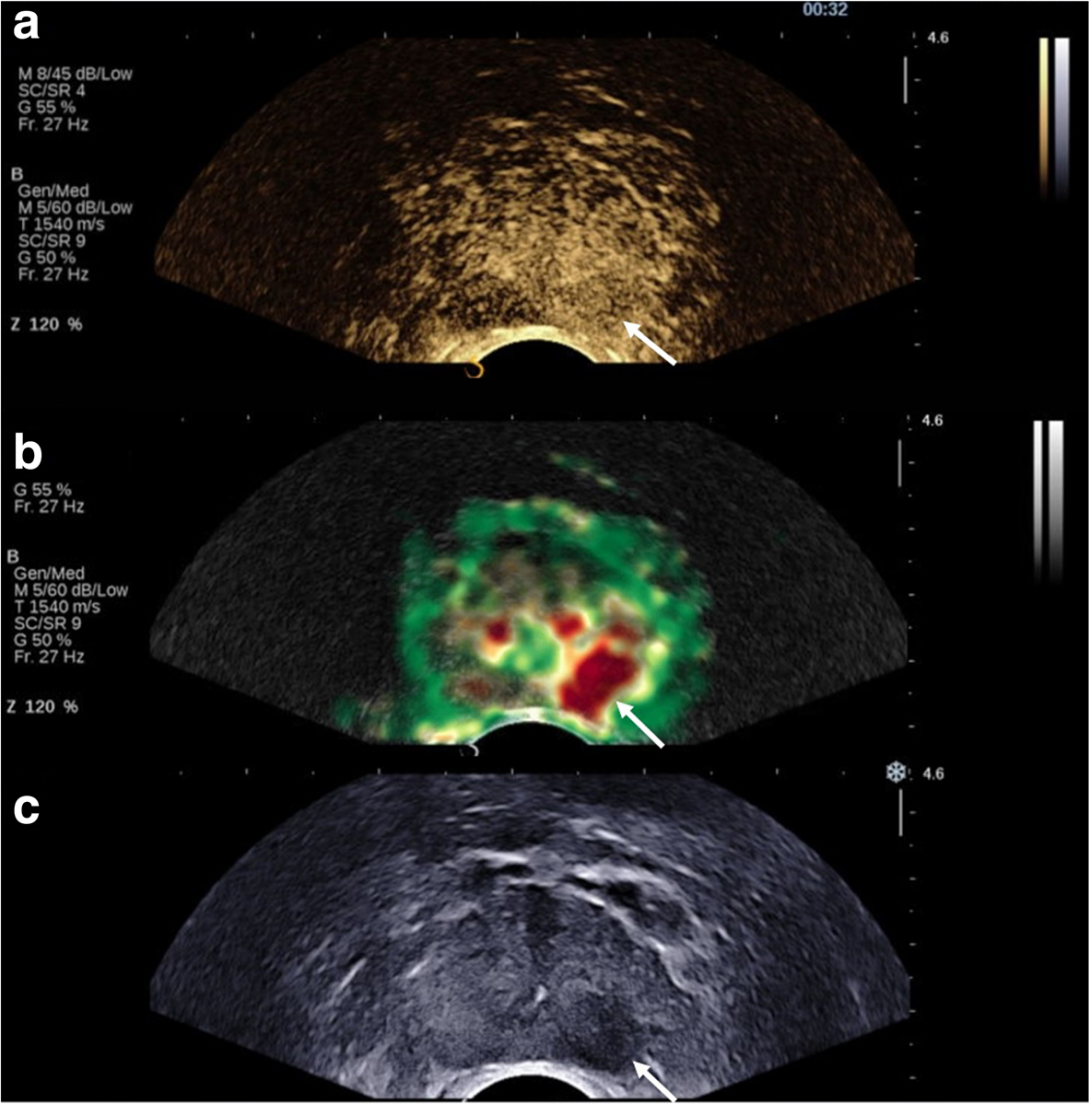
Contrast-enhanced ultrasound and contrast dispersion ultrasound imaging of the prostate. Legend: An area of early contrast enhancement is visible in the left peripheral zone of the prostate in the apical plane (white arrow) (a). Quantitative analysis with the Péclet CUDI parameter demonstrates a suspicious red lesion on the parametric image (white arrow) (b). The suspicious area is also visible as hypo-echogenious lesion on the corresponding greyscale image (white arrow) (c). Radical prostatectomy revealed a pT3a, Gleason 3 + 4 = 7 with tertiary pattern 5 PCa on the left apical side of the prostate
